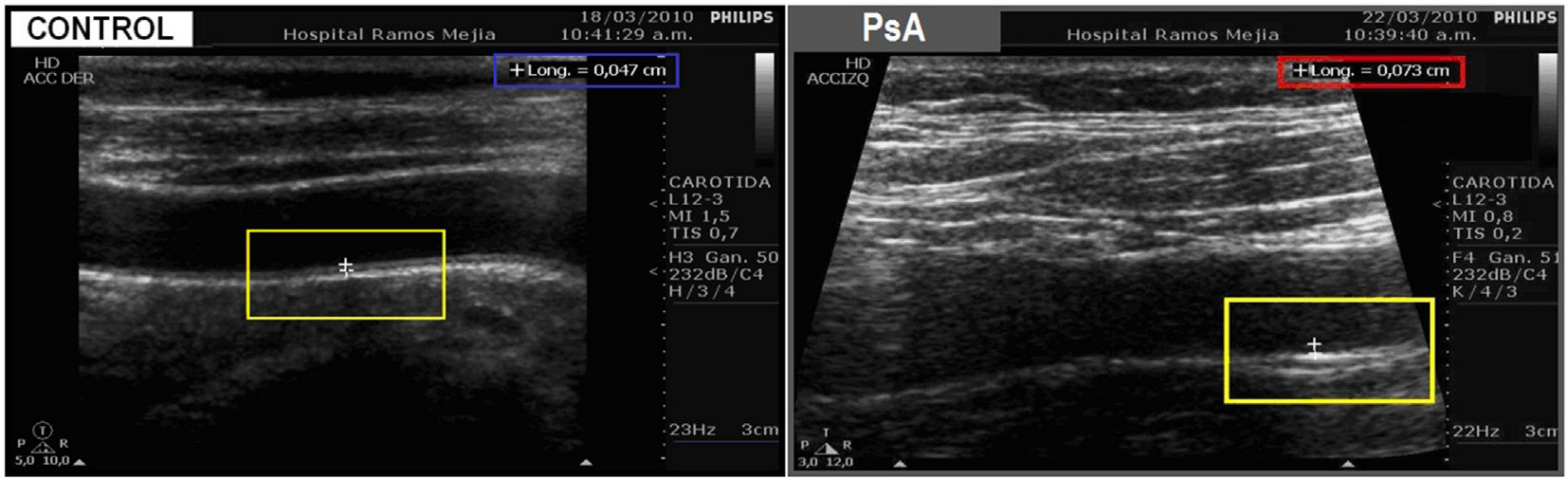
Representative echocardiography images of control patients (left) and PsA patients (right). The left image shows a normal carotid wall with normal intima/media thickness (cIMT = 0.47 mm) in a control patient. Compared with the control image, the right image clearly shows carotid wall thickening (cIMT = 0.73 mm) in a PsA patient.
